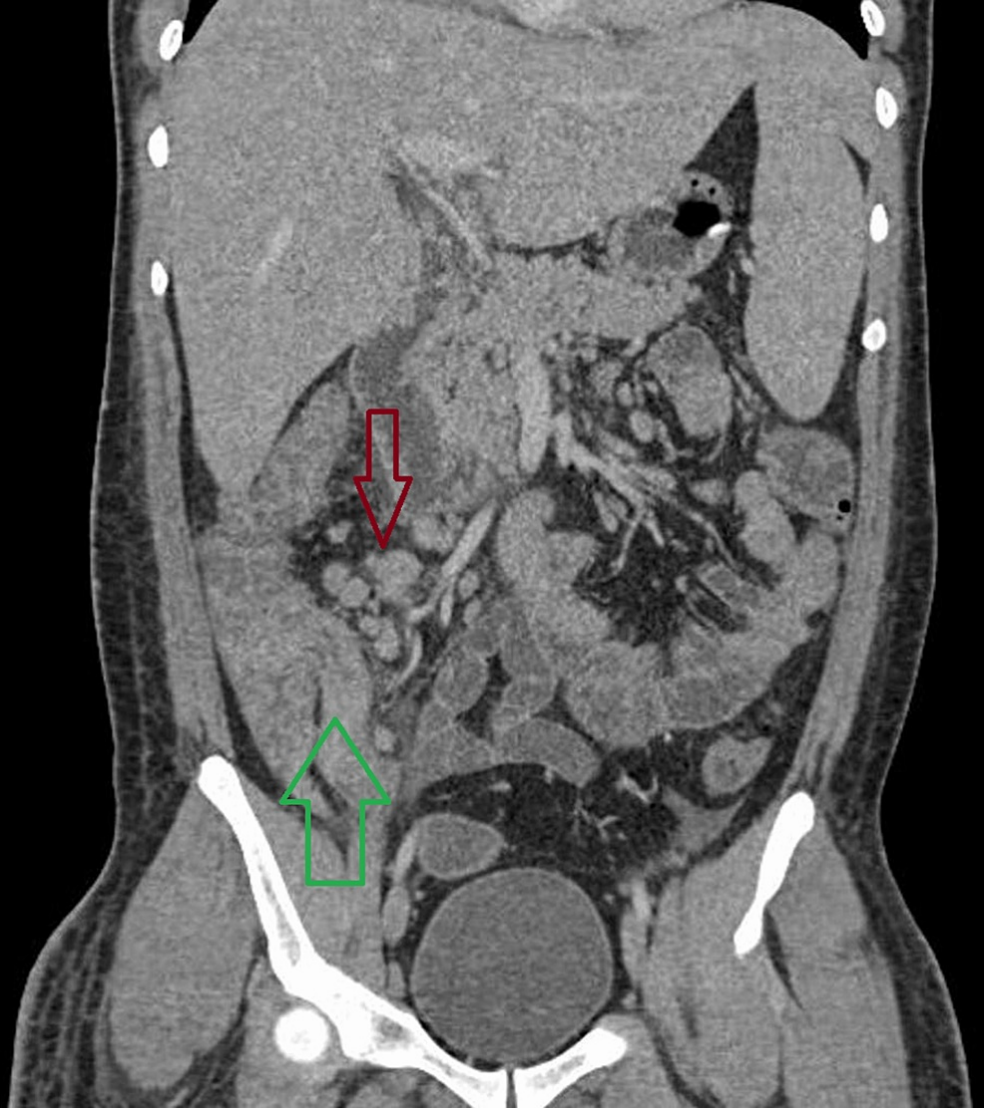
A computed tomography scan of the abdomen (coronal section) shows ileocecal thickening (green arrow) and multiple enlarged mesenteric lymph nodes in the right iliac fossa (red arrow) Circumferential wall thickening involving the terminal ileum, ileocecal junction, cecum, proximal appendix, and ileocecal valve causing narrowing of the ileocecal junction (green arrow) (maximum thickness being 6.5 mm). Extensive surrounding fat stranding is seen in the right iliac fossa. Multiple enlarged mesenteric lymph nodes are also seen (red arrow), the largest being 2.1 X 1.7 cm.
